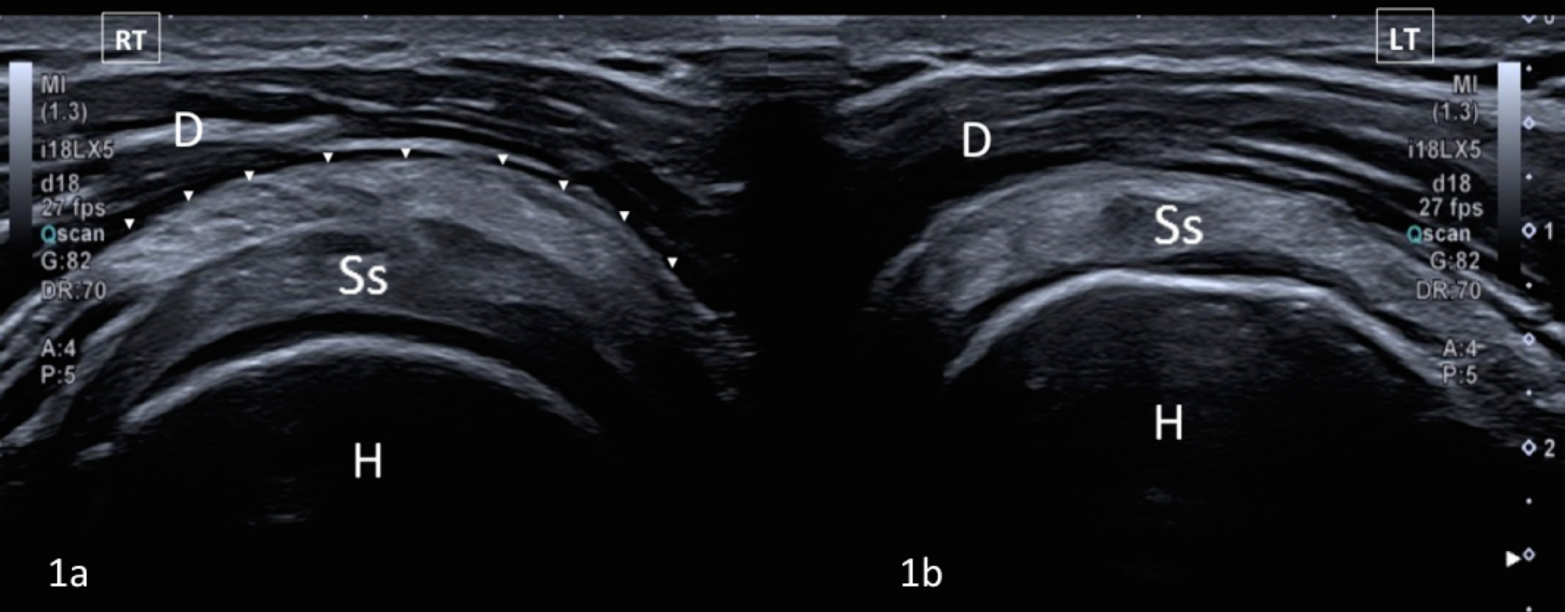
Transverse ultrasound of the anterior shoulder performed using a 15-MHz linear array ultrasound transducer (Aplio i700, Cannon, UK). (a) An image demonstrating an accessory coracobrachialis muscle (arrowheads) in close relation to the subscapularis tendon (Ss) and the deltoid muscle (D). Humeral head indicated by (H). (b) A comparative transverse ultrasound image of the left shoulder of the same patient and demonstrates no accessory coracobrachialis muscle.
